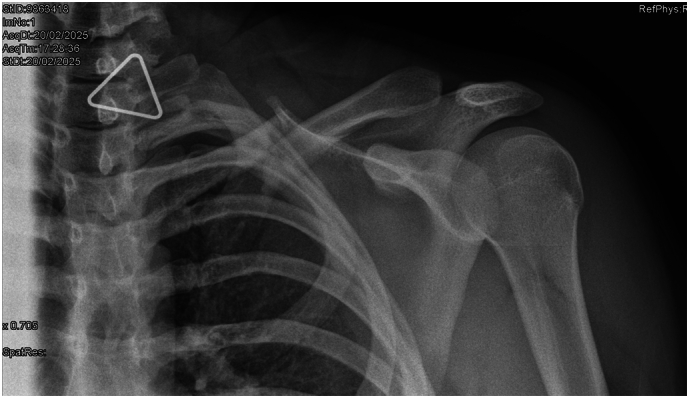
Initial radiograph of left clavicle showing mid shaft clavicle fracture with displacement.
